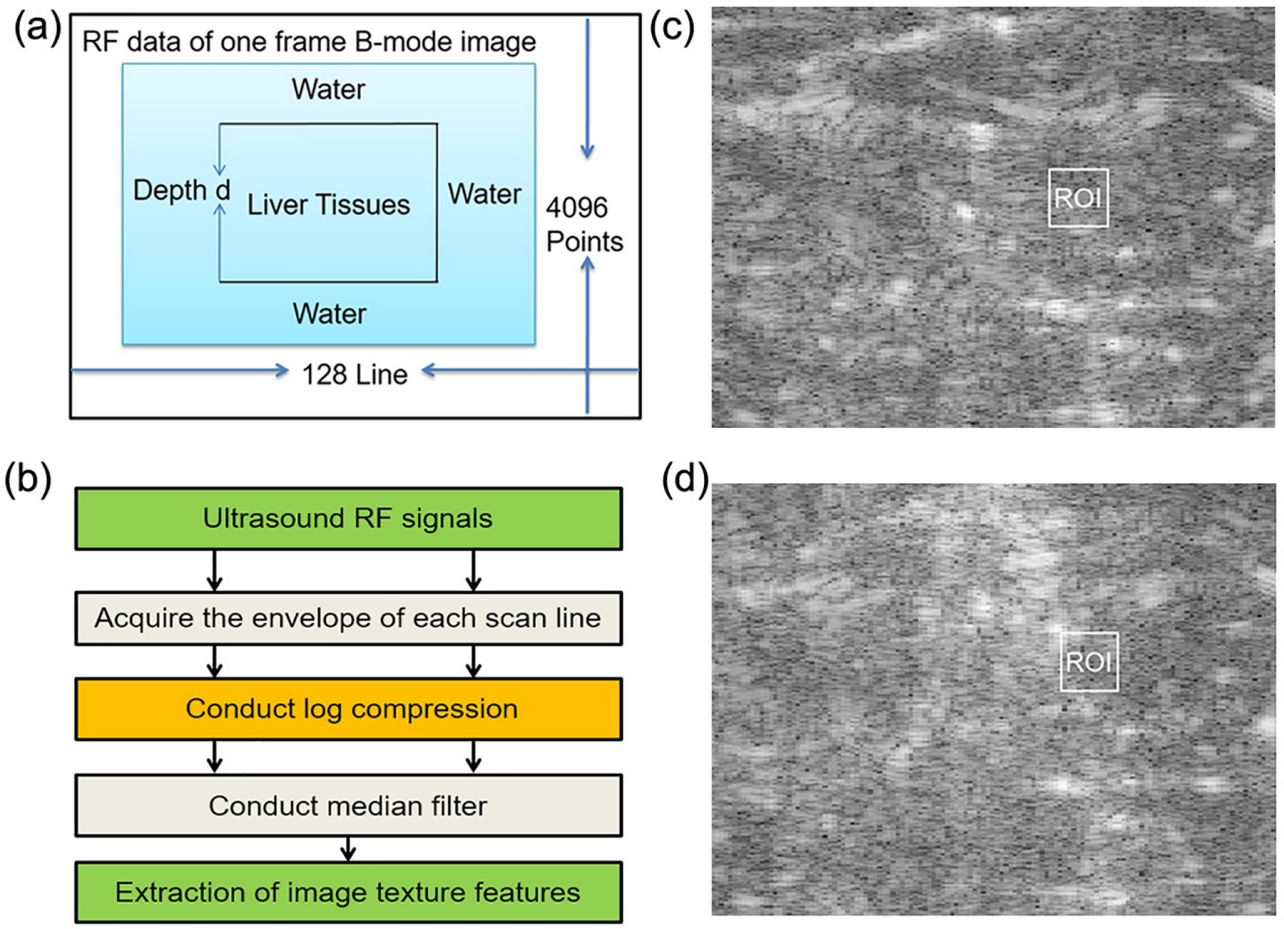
Reconstruction of B-mode images. (a) The ultrasound RF data of one frame B-mode image. (b) Reconstruction B-mode ultrasound image of liver tissue at 20°C during water-bath experiment which represented normal structure, and ROI was selected manually from the images for each sample. (c) Steps for image reconstruction from ultrasound RF signals. (d) Reconstruction B-mode ultrasound image of liver tissue at 60°C during water-bath experiment which represented coagulated structure, and ROI was selected manually from the images for each sample.
